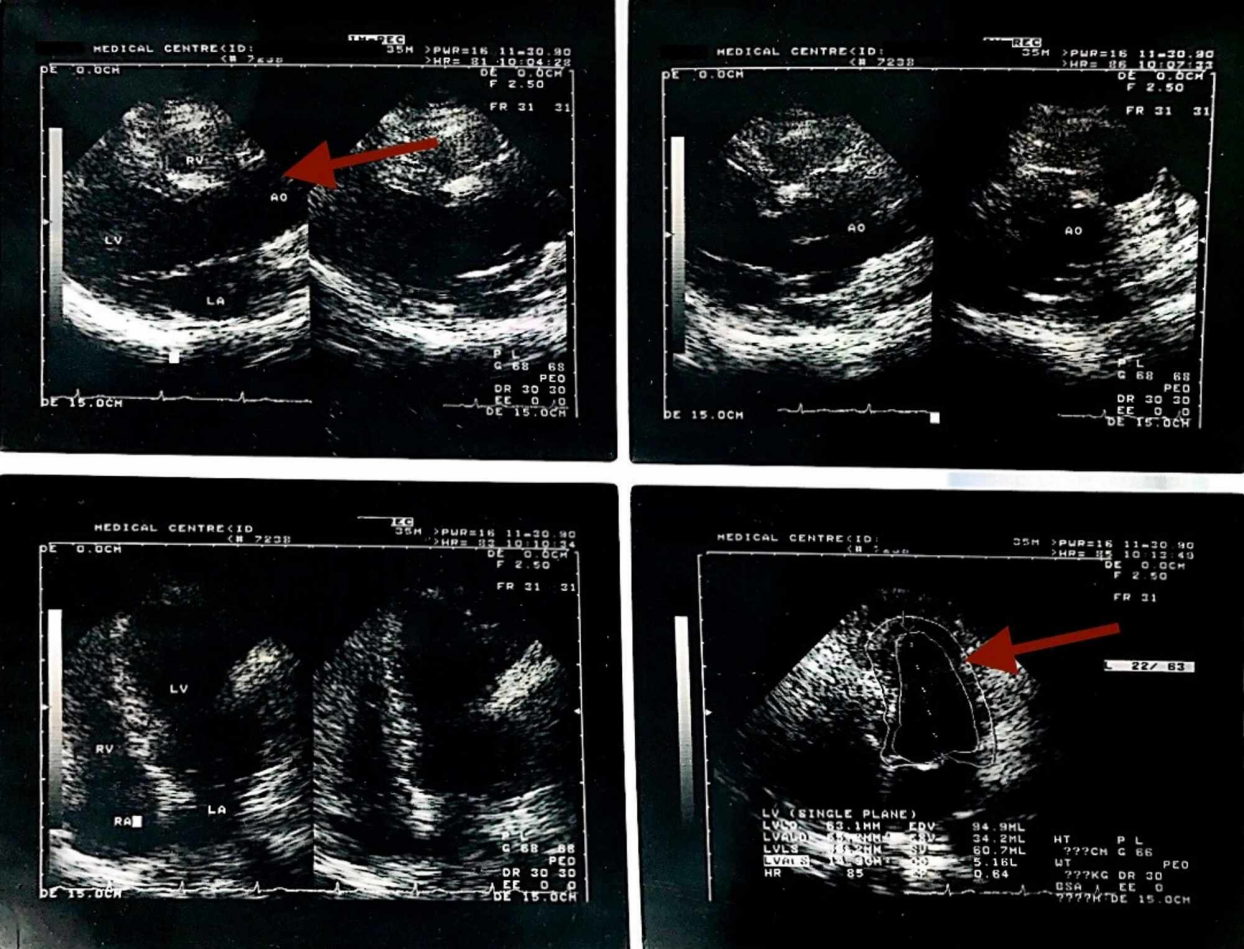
Transthoracic echocardiographic images of the heart - Nov 1990.
